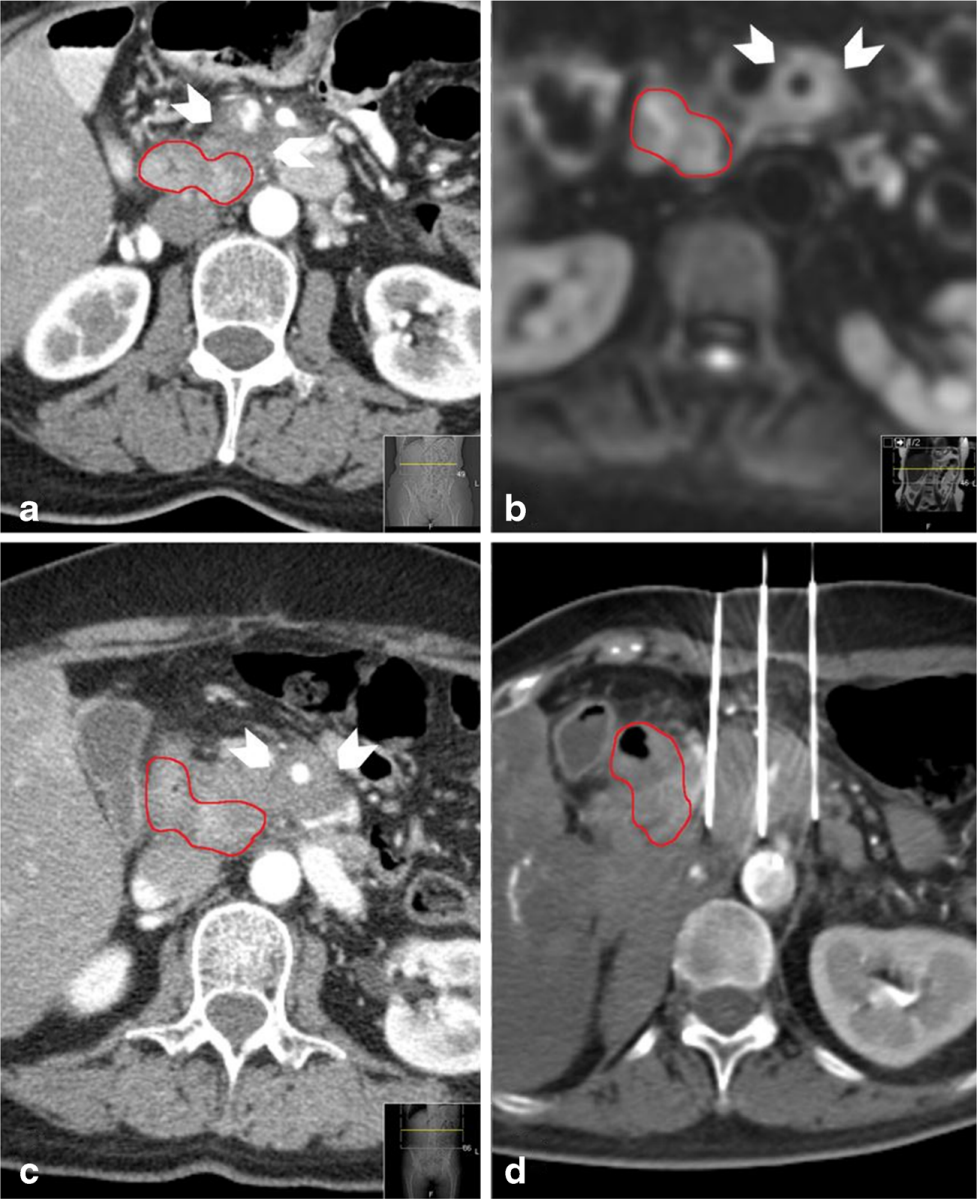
The development of a local recurrence. *Red line* = duodenum. a CeCT pre-IRE showing the initial tumour (*white arrowheads*) that was treated with IRE (b) MR DWI-b800 6 weeks post-IRE showing new hyperintensity around the superior mesenteric artery (*white arrowheads*) (c) CeCT 4 months post-IRE showing evident local recurrence (*white arrowhead*) (d) re-IRE of the local recurrence
